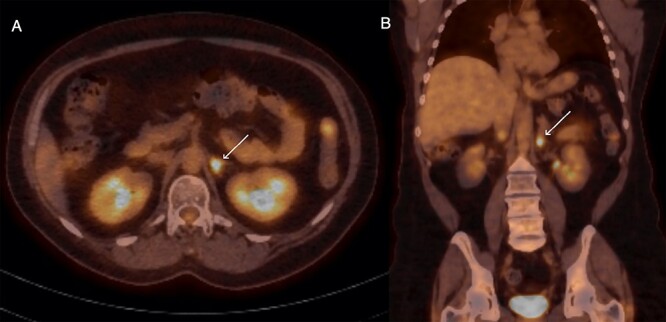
Axial (A) and coronal (B) sections of FDG-PET demonstrating glucose-avid lesion in the left adrenal (arrow).
